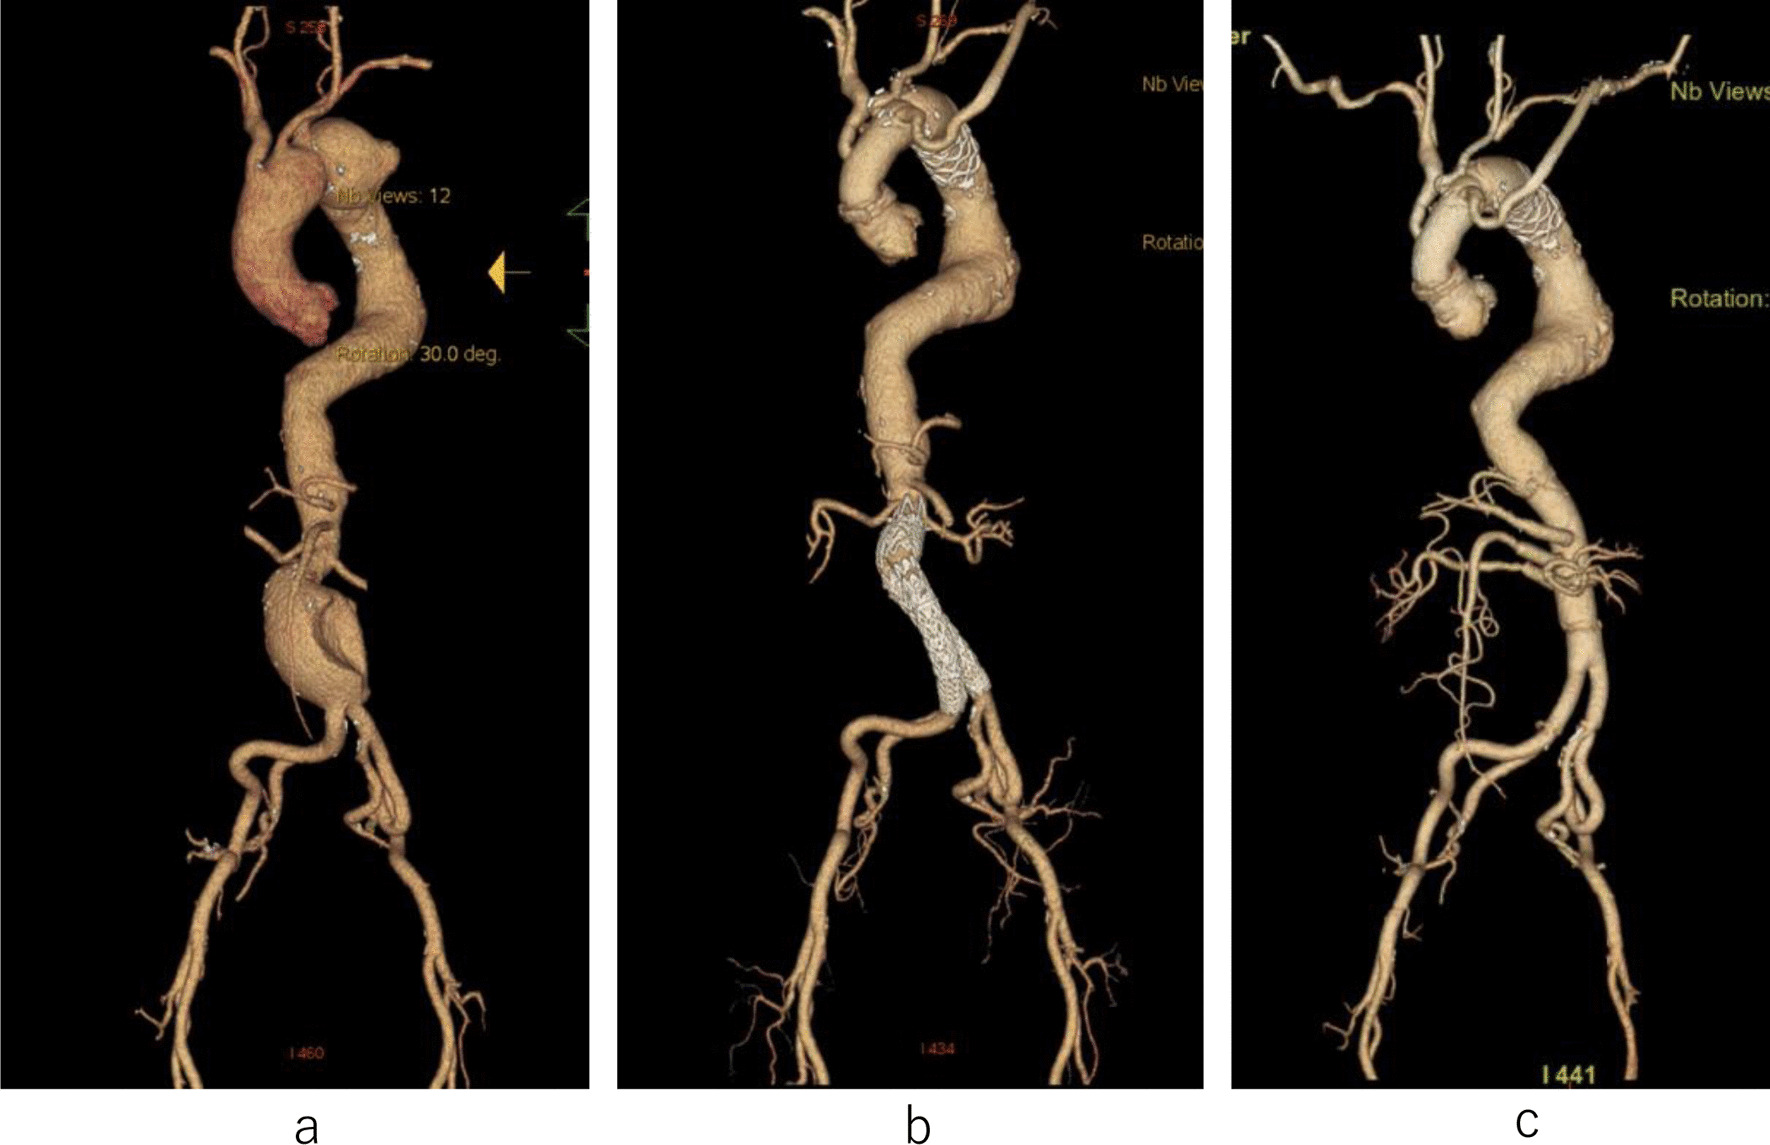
3D-CTA images of case 4. a The thoracic and abdominal aortic aneurysms in case 4; b The abdominal aorta after endovascular aneurysm repair and total arch repair with the frozen elephant trunk procedure; c the dilated descending aortic aneurysm after thoracoabdominal replacement
